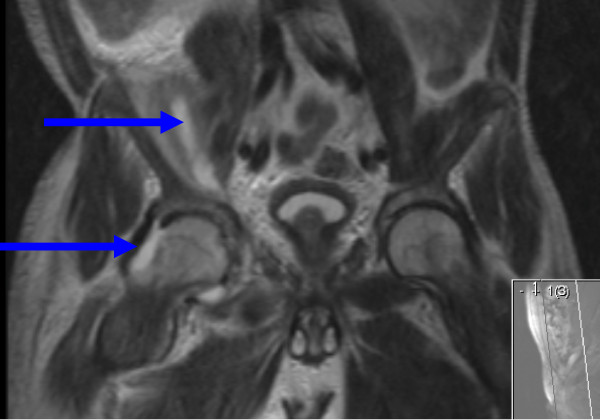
A T2 weighted MRI image of the pelvis in the coronal plane. This image demonstrates an area of high signal around the right iliacus and psoas muscles. It also shows evidence of fluid in the hip joint.
